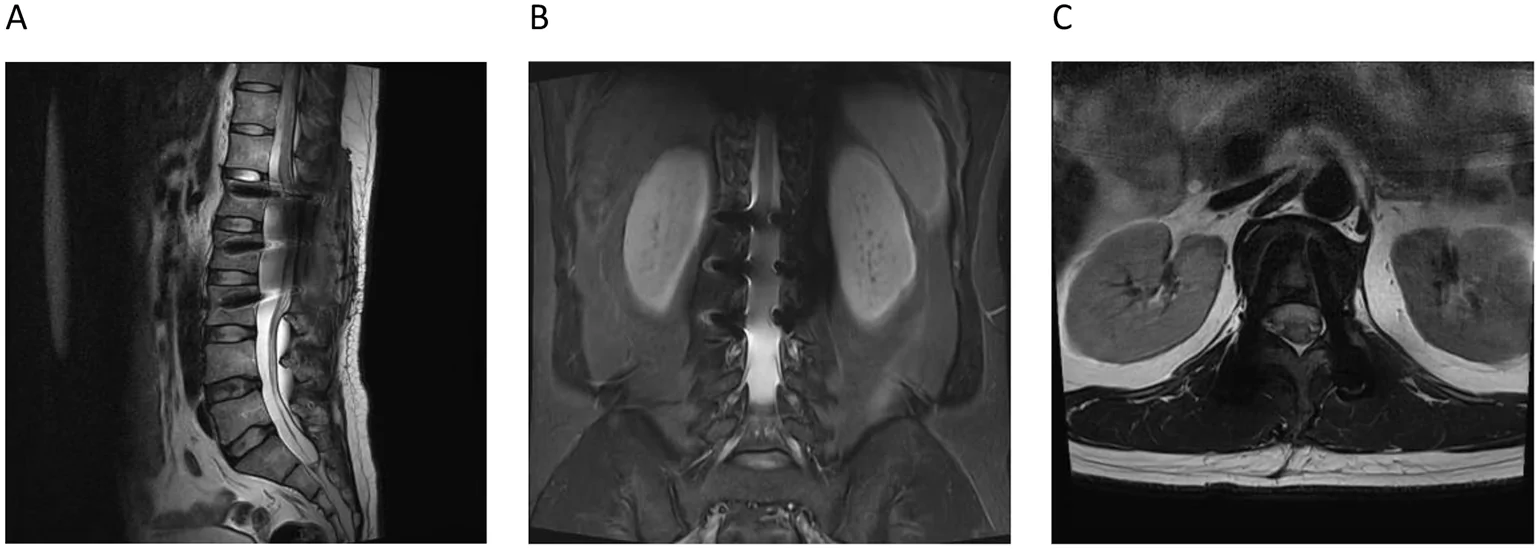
MRI contrast-enhanced follow-up examination at six months postoperatively. (A) Contrast-enhanced sagittal view; (B) Contrast-enhanced coronal view; (C) Contrast-enhanced axial view showing no obvious abnormal enhancement. Degenerative changes and bulging are observed at the L4/L5 and L5/S1 intervertebral discs.
